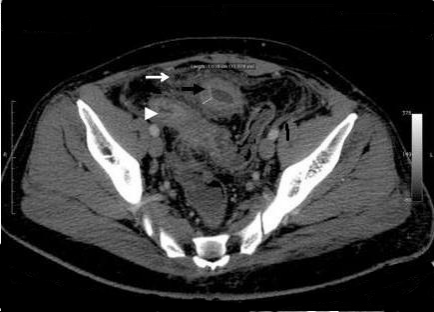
Preoperative abdominal CT scan. Shows a small bowel loop with a thickened wall and narrow lumen with proximal bowel dilation (black arrow). Increased density of the adjacent mesenteric fat (white arrow), as well the normal distal ileum (head arrow) can also be seen.
